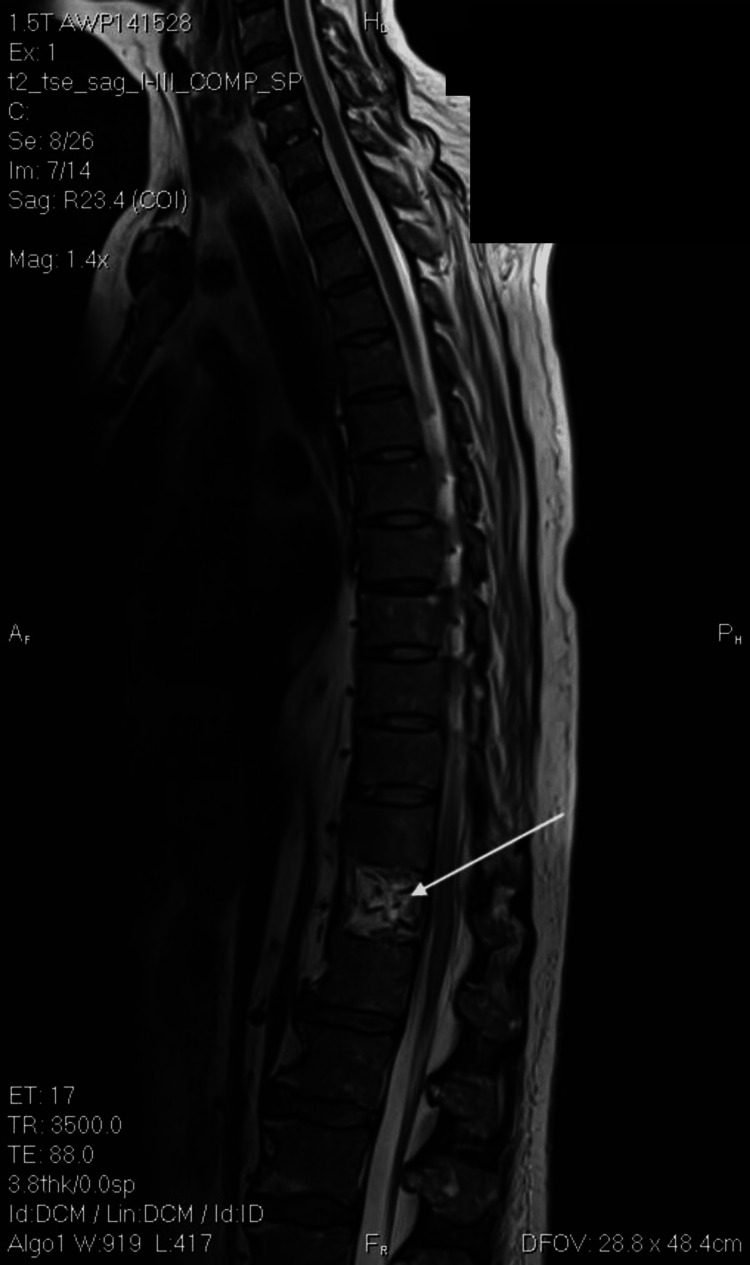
Magnetic resonance imaging of the spine in sagittal view. The white arrow indicates compression fracture.
